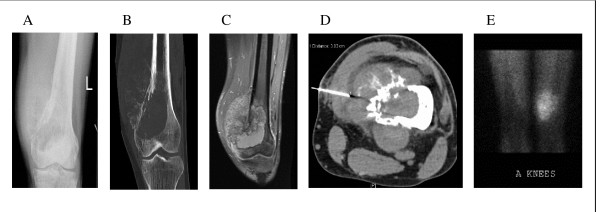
Imaging features of osteosarcoma. Panel A is a plain radiograph demonstrating a lytic destructive lesion with malignant new bone formation involving the medial aspect of left distal femur. Panel B is a coronal CT image showing typical periosteal new bone formation with cortical destruction associated with a lytic bony lesion involving the left distal femur. Panel C is an enhanced MR image demonstrating an eccentric left distal femoral metaphyseal mass with a large extra-osseous component. Panel D is an axial image of a CT-guided biopsy sampling of the extra-osseous mass. Panel E is an image from a thallium bone study showing uptake overlying the distal left femur indicative of high grade tumour at this site.
